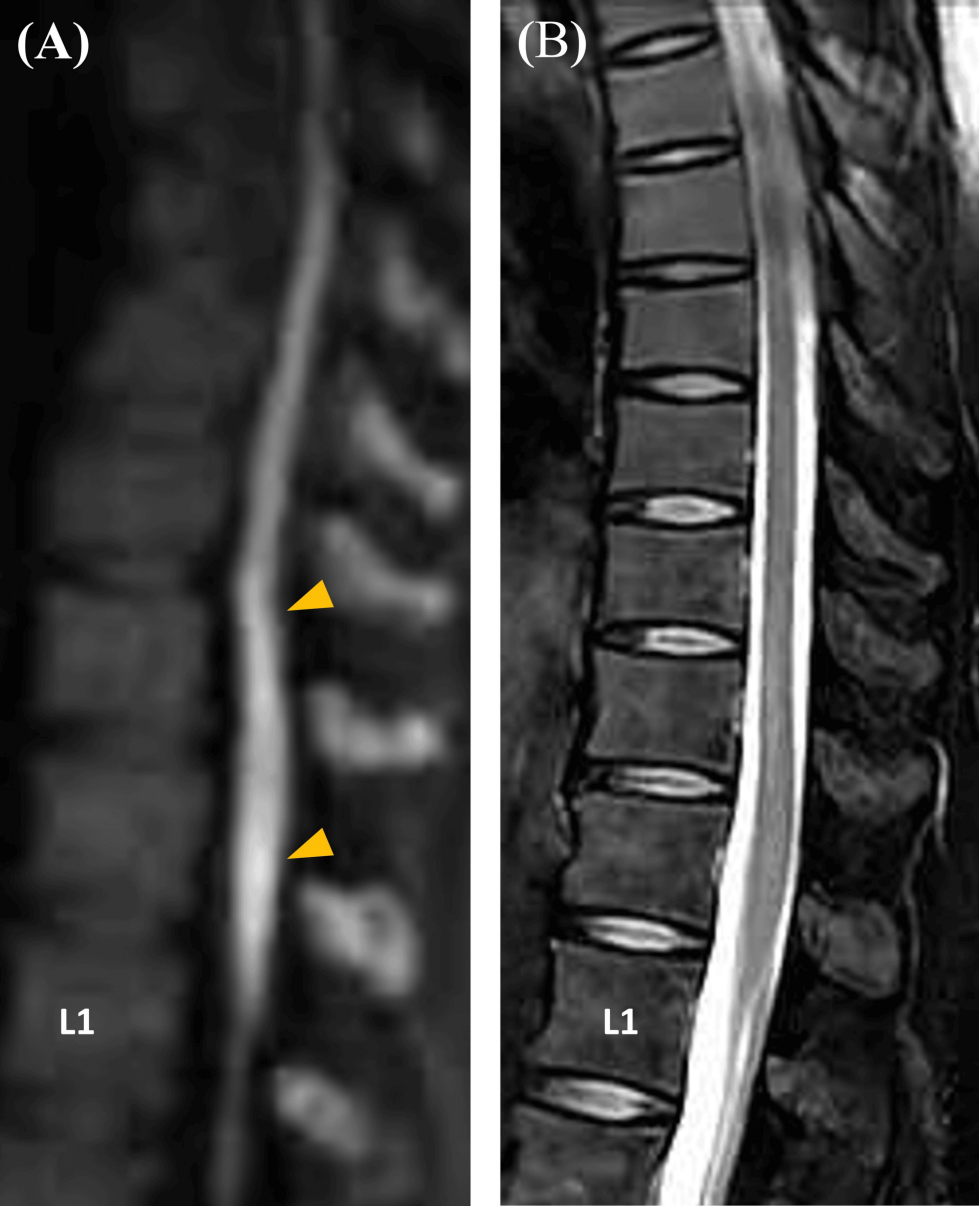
The results of thoracic magnetic resonance imaging (MRI) on diffusion-weighted and T2-weighted imaging on admission. A: diffusion-weighted thoracic MRI showing hyperintensities in the spinal cord at the Th11 and Th12 levels; B: T2-weighted MRI showing no hyperintensity in the spinal cord
